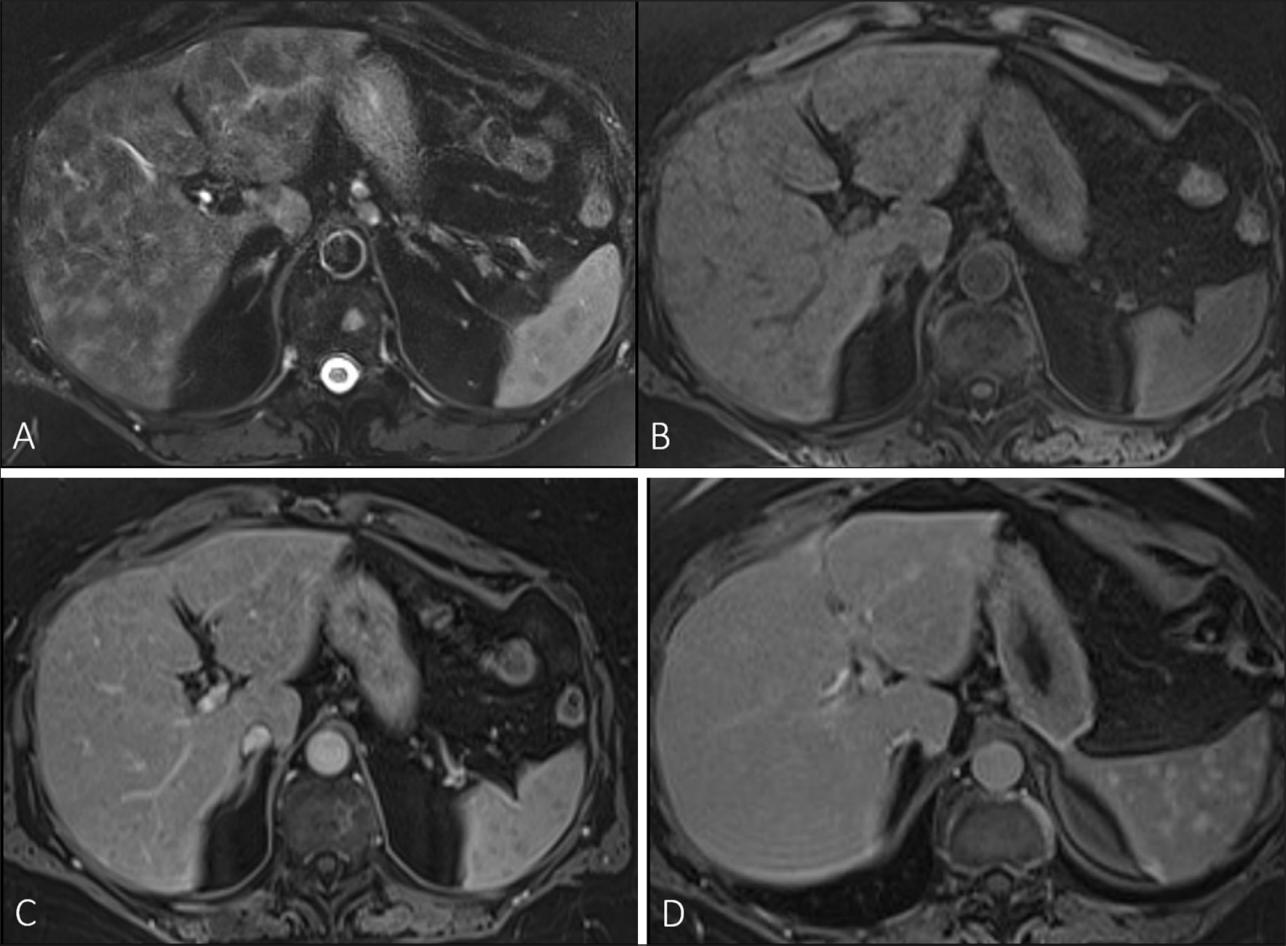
MRI of the upper abdomen at time of the initial diagnosis. Axial fat
